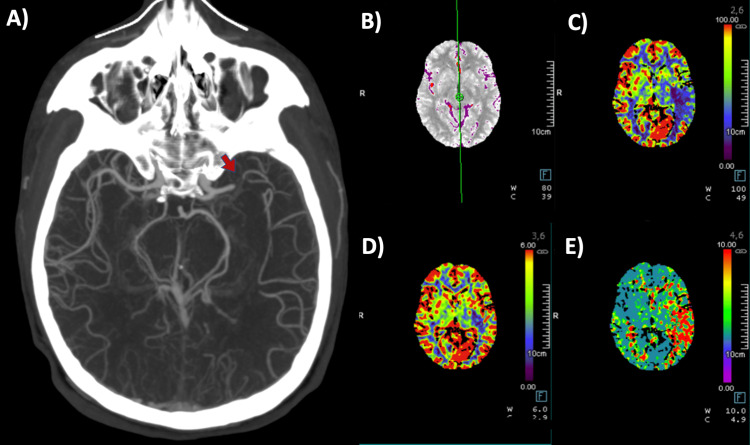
Brain CT angiography images The images demonstrate left MCA occlusion in the M1 segment (arrow) (A); reference perfusion processing (B), with hypoperfusion area with low blood flow (C); and low blood volume (D); and an increase in median transit time (E) in cerebral perfusion maps CT: computed tomography; MCA: middle cerebral artery
